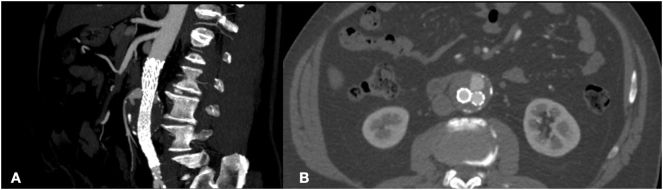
CT-angiography at 2 months after EVAR showing type 2 endoleak fed from inferior mesenteric artery in sagittal plane (A) and traversal plane (B).
